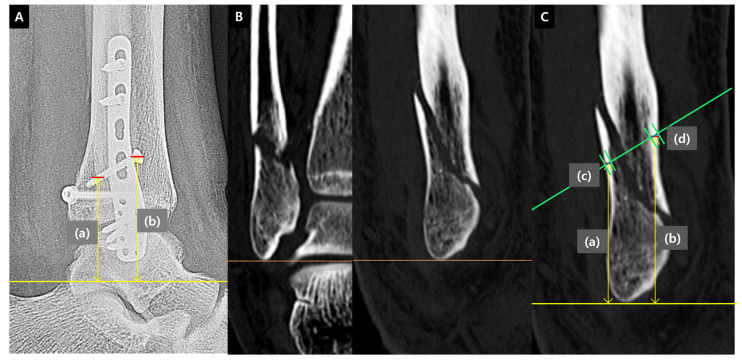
Measurement technique for anterior and posterior cortical thickness around the interfragmentary screw fixation level. (A) On postoperative ankle radiographs, a horizontal reference line was drawn through the tip of the lateral malleolus. The vertical distances from this reference line to the points where the interfragmentary screw crossed the posterior (a) and anterior (b) cortices of the fibula were measured. (B) On preoperative computed tomography (CT) images, the reference level corresponding to the lateral malleolar tip was identified using a cross-reference tool. (C) The distances measured on postoperative radiographs were transferred to the sagittal CT image to estimate the screw-cortex intersection levels. The posterior (c) and anterior (d) cortical thicknesses were then measured at these estimated levels.
